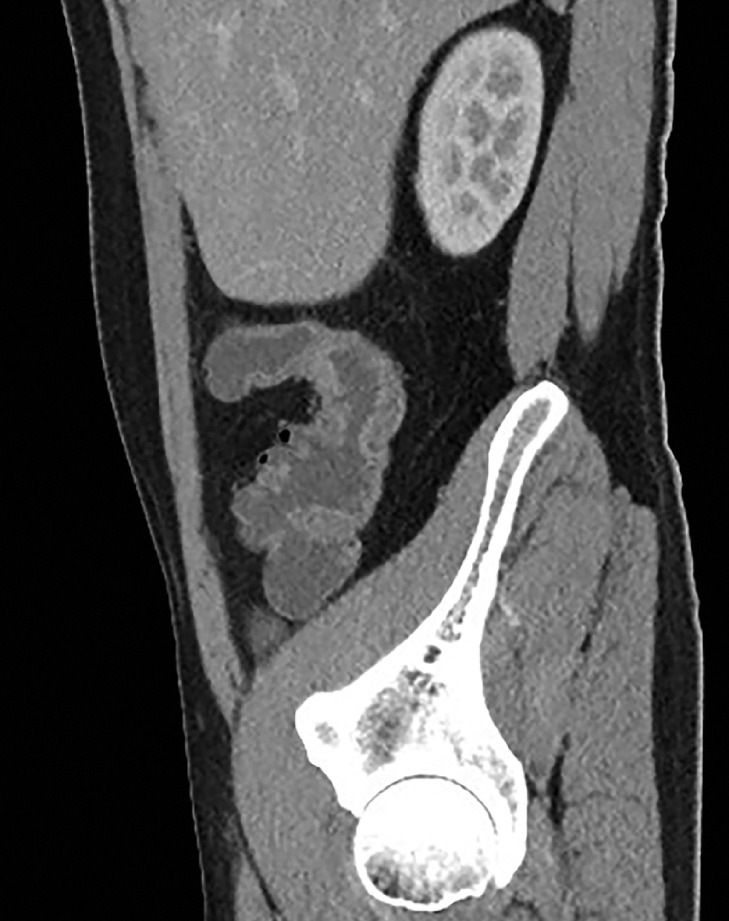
Computed tomography showed severe stricture of the ascending colon in Crohn’s disease.
